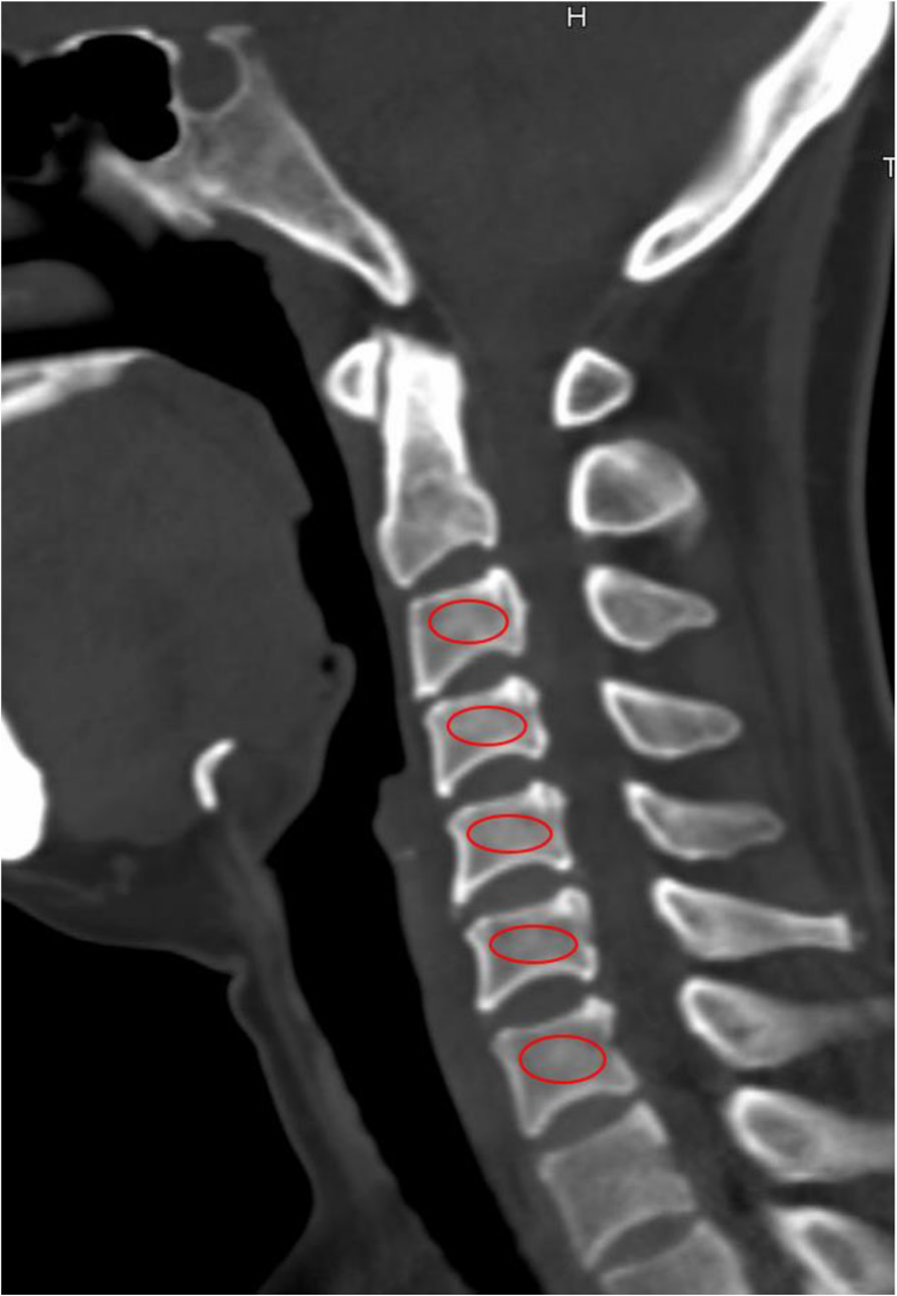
Measurement of HU values in the lateral CT scan reconstruction images
